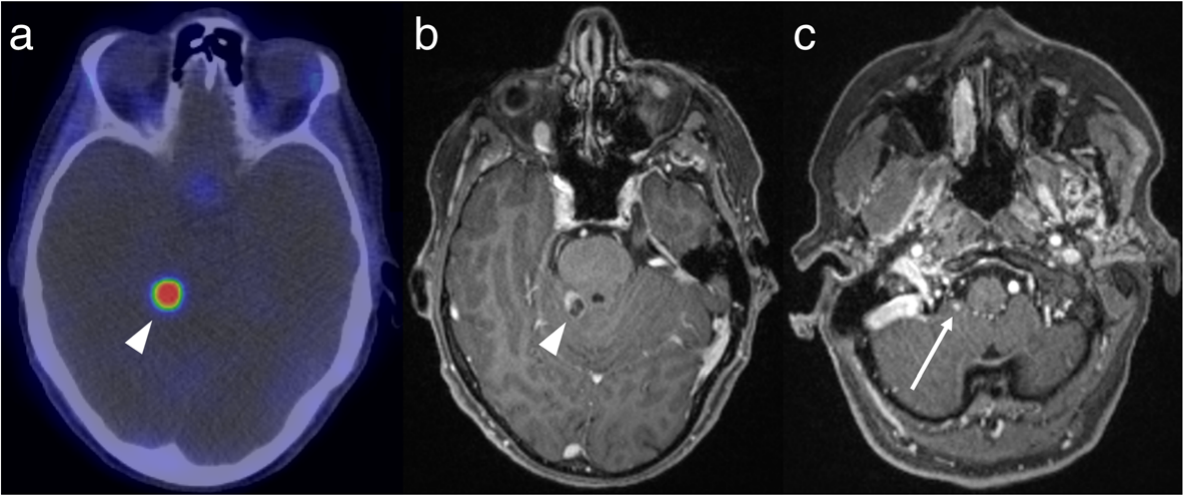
GaTate-PET (a) in a patient with metastatic NET identifies a lesion in the posterior fossa (arrowhead). Given the proximity to the tentorium cerebelli, both an incidental meningioma and a NET metastasis are in the differential. The mixed solid and cystic appearance on the post-contrast MRI (b) confirms a metastasis. MRI also demonstrates a smaller enhancing focus more inferiorly in the posterior fossa (c), consistent with a further NET metastasis
